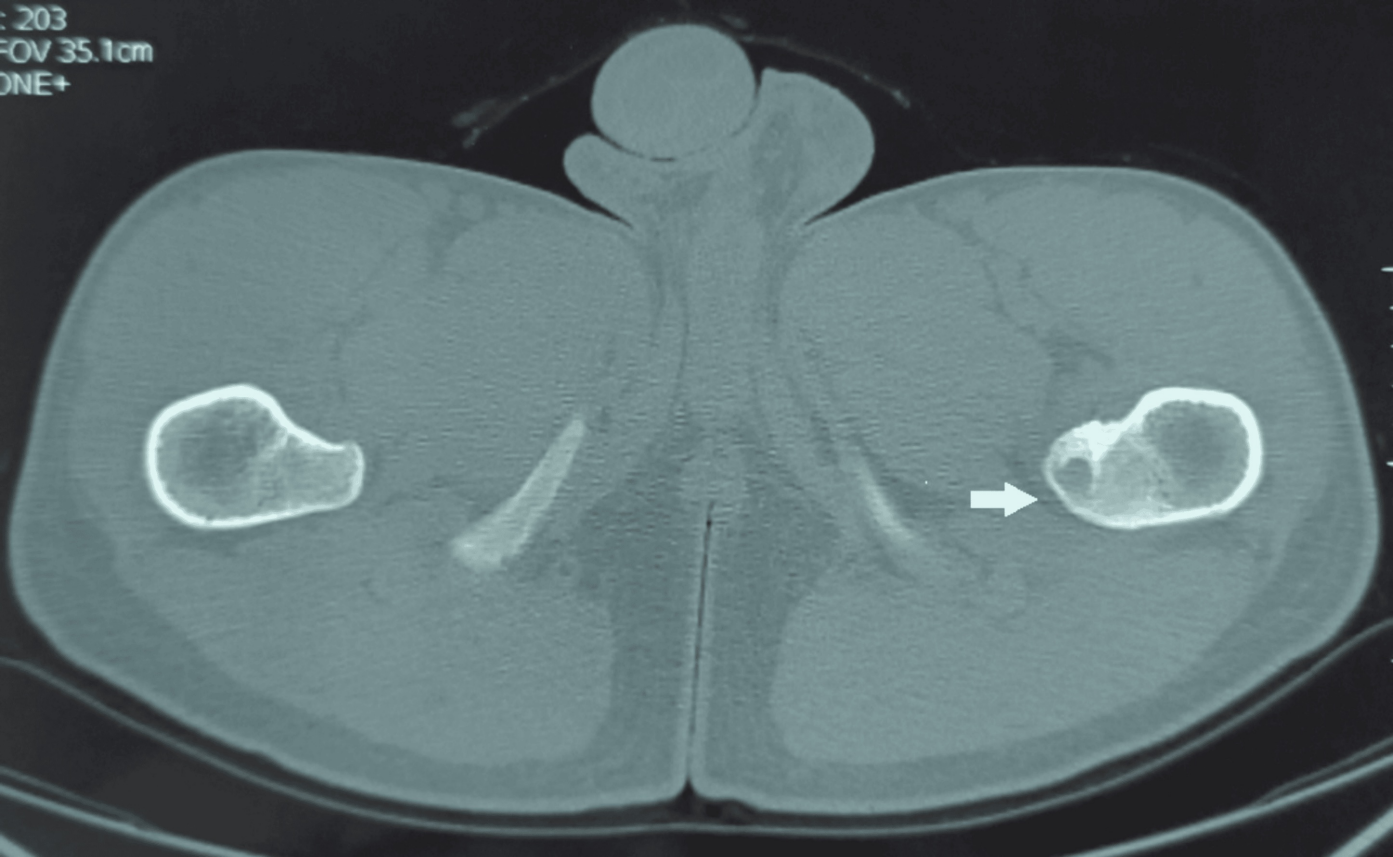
A non-contrast CT scan of the pelvis in the axial section showing the lesion of the left lesser trochanter.
